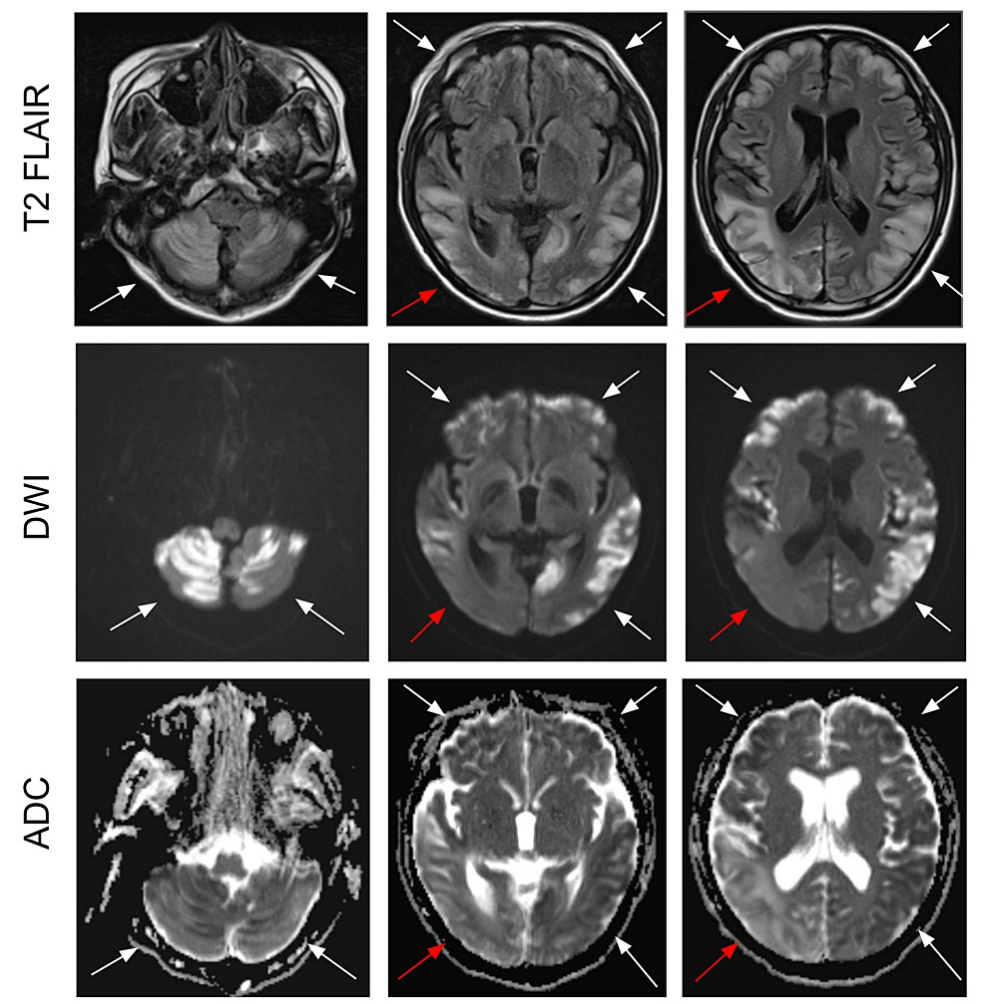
Postoperative MRI brain Significant worsening with progression of bilateral supra and infratentorial variables extensive increased FLAIR and T2-weighted with high diffusion and low ADC signal. Findings are most consistent with ischemic changes with multiple bilateral new (white arrows) and previous right parietal (red arrow) infarctions.
